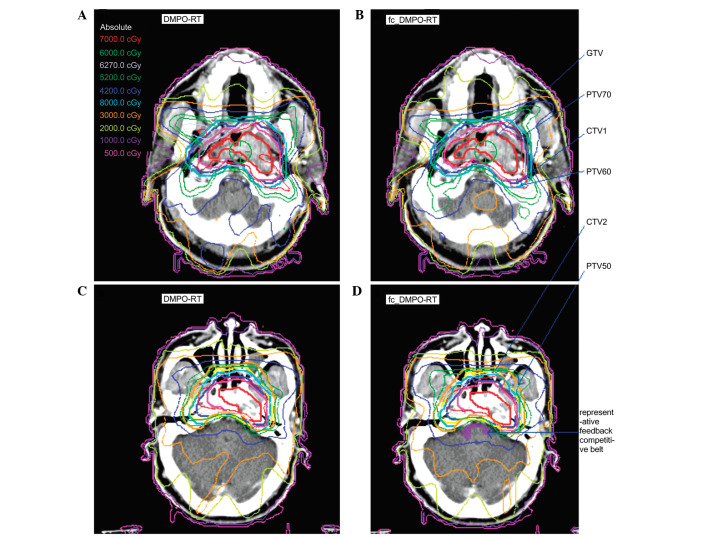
Axial computed tomography images comparing representative isodoses between two planes. (a) DMPO-RT, (b) fc_DMPO-RT (c) DMPO-RT and (d) fc_DMPO-RT. fc_DMPO-RT was better than DMPO-RT, and the representative feedback competitive belt is marked. GTV, gross tumor volume; DMPO-RT, direct machine parameter optimization radiotherapy method; fc_DMPO-RT, feedback constraint DMPO-RT method; PTV, planned target volume; OAR, organs at risk; CTV, clinical target volume.
